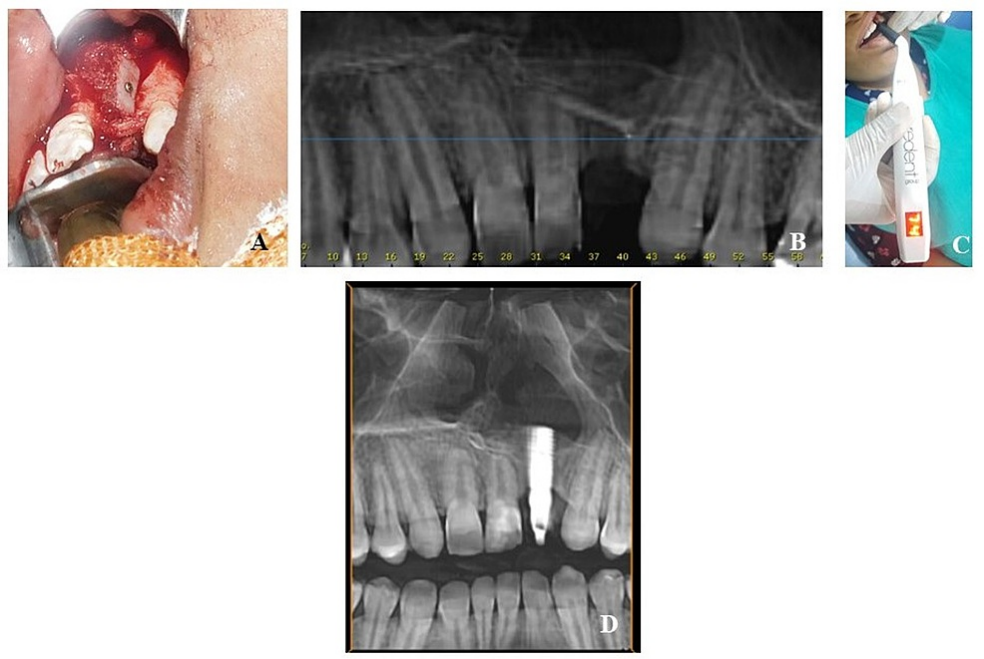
A: Iliac graft stabilized in defect using titanium screw during tertiary grafting. B: Cone-beam computed tomography scan recorded six months after grafting. C: Primary ISQ recording. D: CBCT recorded after implant placement. CBCT, cone-beam computed tomography.
